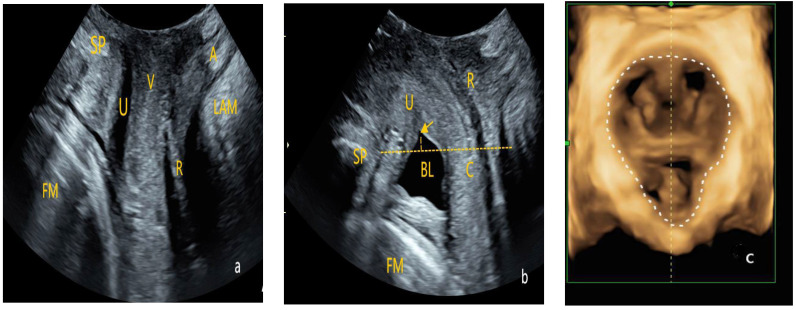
Transperineally pelvic floor ultrasound examination. (a) Two-dimensional pelvic floor ultrasonographic image in the mid-sagittal plane obtained at rest (FM: fetal head; SP: pubic symphysis; U: urethra; V: vagina; A: anal canal; R: rectal ampulla; LAM: levator ani muscle). (b) Two-dimensional pelvic floor ultrasonographic image during maximum Valsalva maneuver to assess bladder protrusion and urethral funneling (BL: bladder; Arrow: proximal urethra showing funneling; Yellow dashed line: a horizontal line drawn through the posterior-inferior border of the pubic symphysis). (c) Four-dimensional sonographic image of the levator ani muscle hiatus (White dashed line: the boundary of the hiatal area, which is the area enclosed by the pubic rami, the pubic symphysis, and the medial border of the puborectal muscle).
